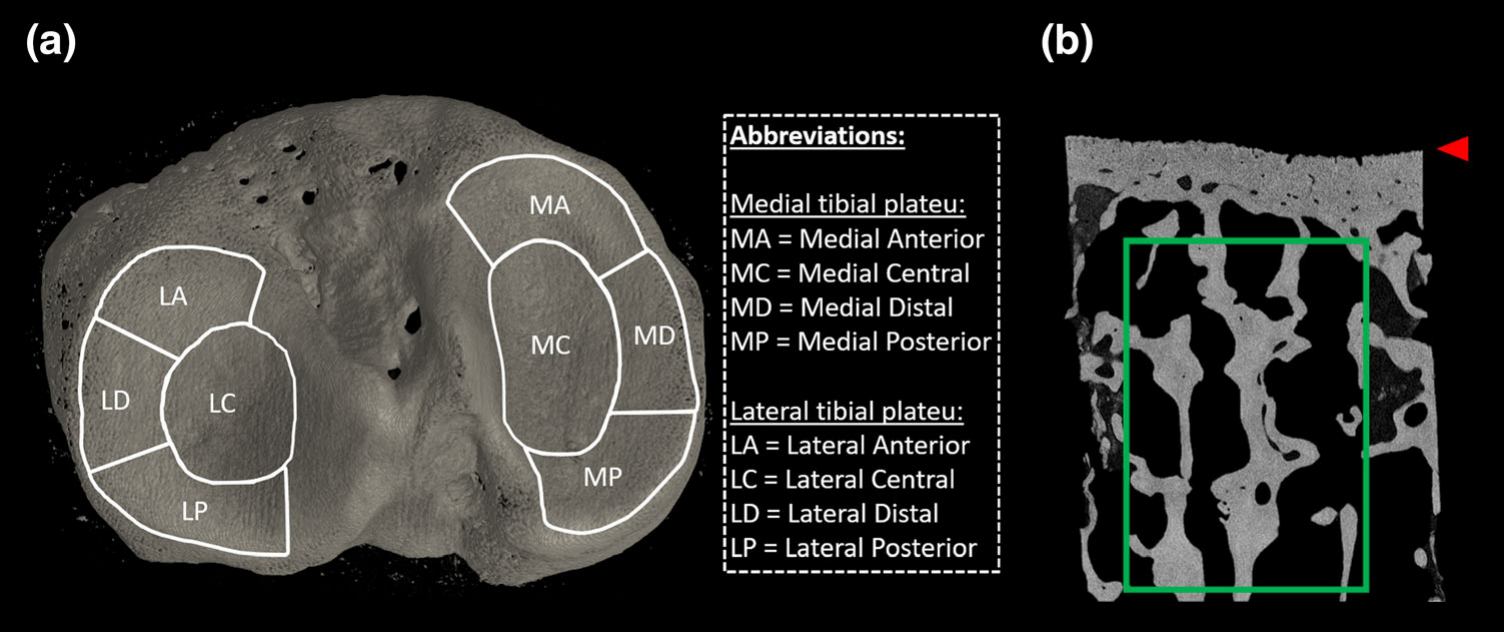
Core extraction and VOI selection. (a) 8 areas from tibial plateau from which the osteochondral cores were extracted. (b) Sagittal slice of *µ*CT imaged subchondral bone core on which the trabecular bone VOI (green rectangle) and calcified cartilage—articular cartilage interface (red arrow) are marked.
